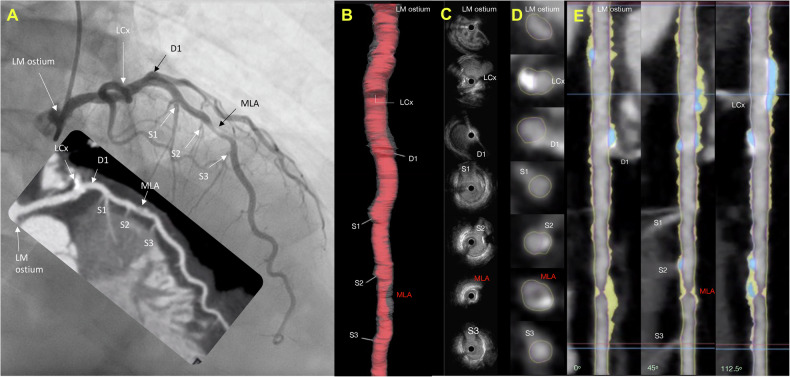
Example of multi-modality co-registration. First, the intravascular ultrasound (IVUS) core laboratory created a detailed map of anatomical landmarks (coronary ostium, side branches, calcifications, and stenoses) common to the angiography (A) and IVUS pullback (B, C). Next, the same landmarks were identified on coronary computed tomography angiography (CCTA) images (inset in A and D, E). The distance between the most proximal and distal co-registered landmarks defined the analysis segment, irrespective of the plaque burden. D (diagonal branches), LM (left main coronary artery), LCx (left circumflex artery), MLA (minimum lumen area), S (septal branches)
